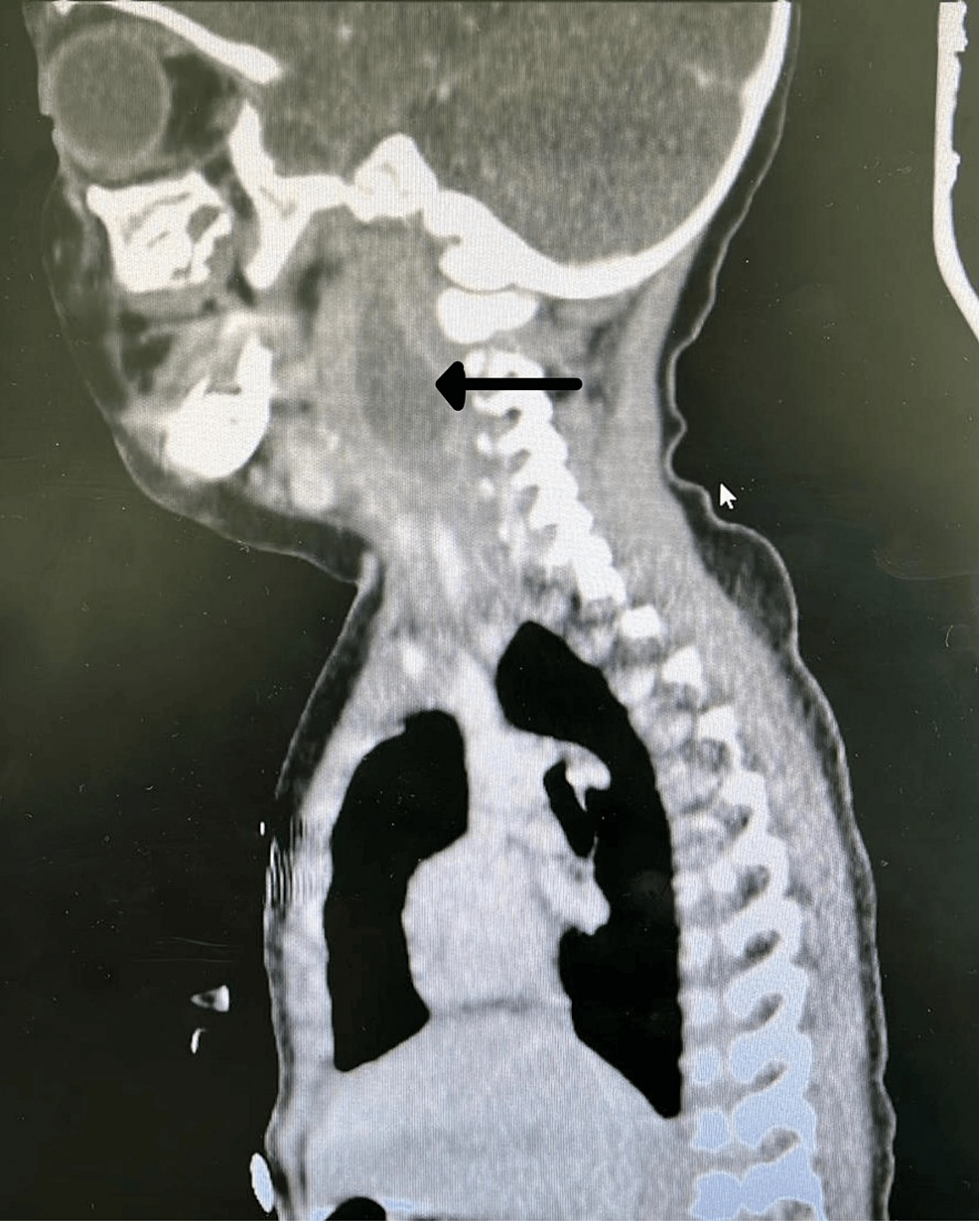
Axial view of CT scan showing right-sided retropharyngeal and parapharyngeal abscess measuring about 27 x 33 x 12 mm in maximum dimensions that connects to the right side of oropharynx at C3 level with marked compromise of airway and multiple enlarged lymph nodes. CT: computed tomography; C3: cervical spine 3.
